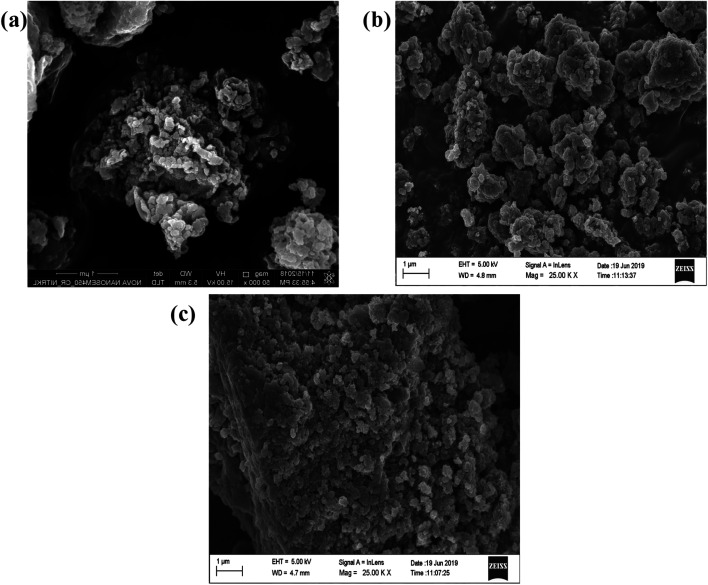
FESEM images of (a) CT, (b) B-CT and (c) S-CT.
